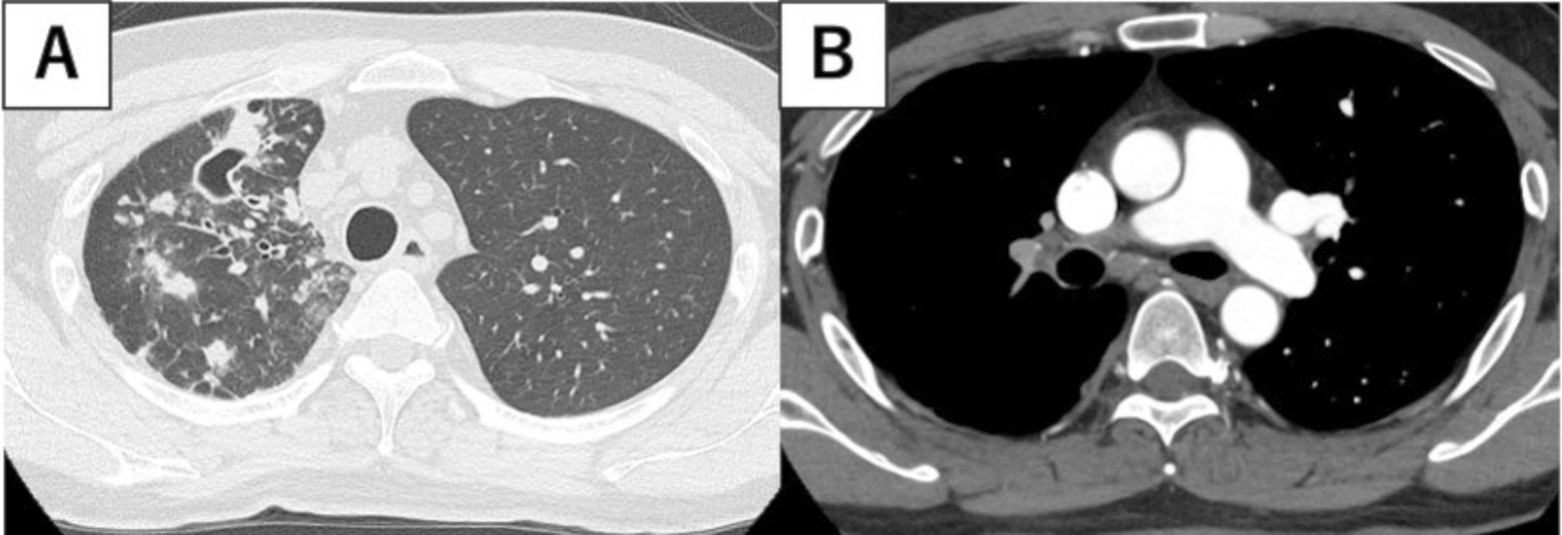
Radiographic images taken 2 years after the first examination. (A) CT showing new nodules with cavities in the upper right lobe. (B) CT showing a residual thrombus in the main trunk of the right pulmonary artery.
